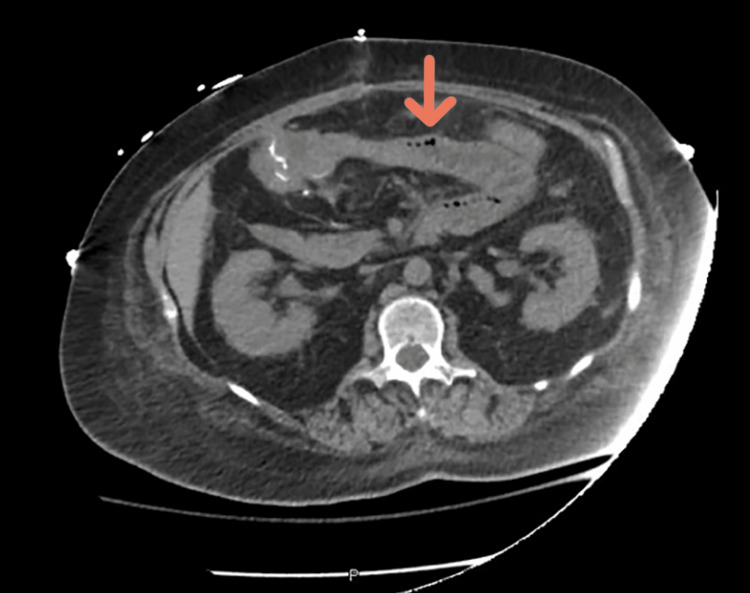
CT scan of the abdomen showing Pneumatosis intestinalis
